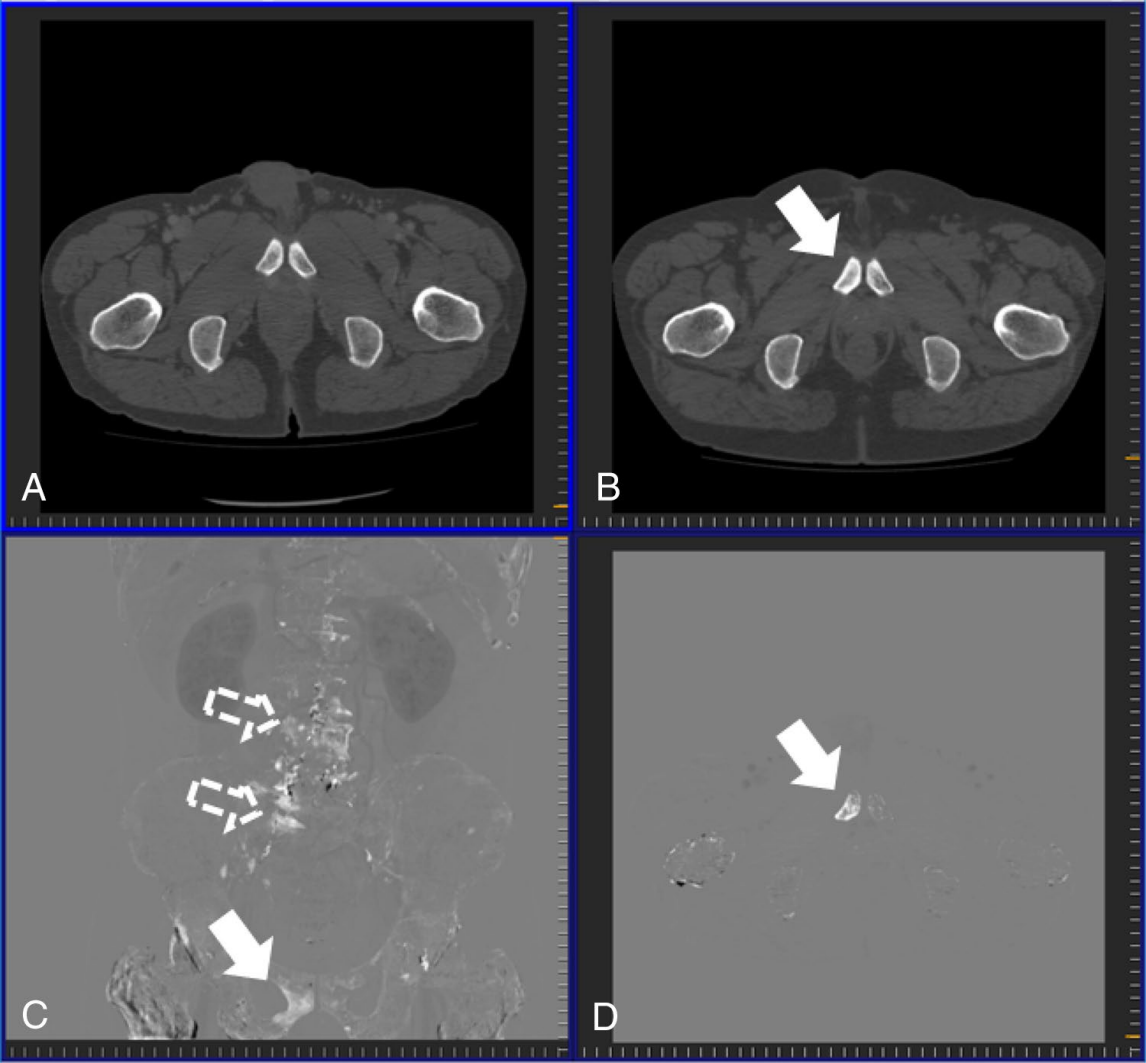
Osteogenic metastasis in the right pubis (arrow) accompanied by degenerative changes (outlined arrow) in a 59-year-old male patient with prostate cancer. (A) Previous computed tomography (CT) image; (B) current CT image; (C) temporal subtraction (TS) image; (D) projection image of TS images.
